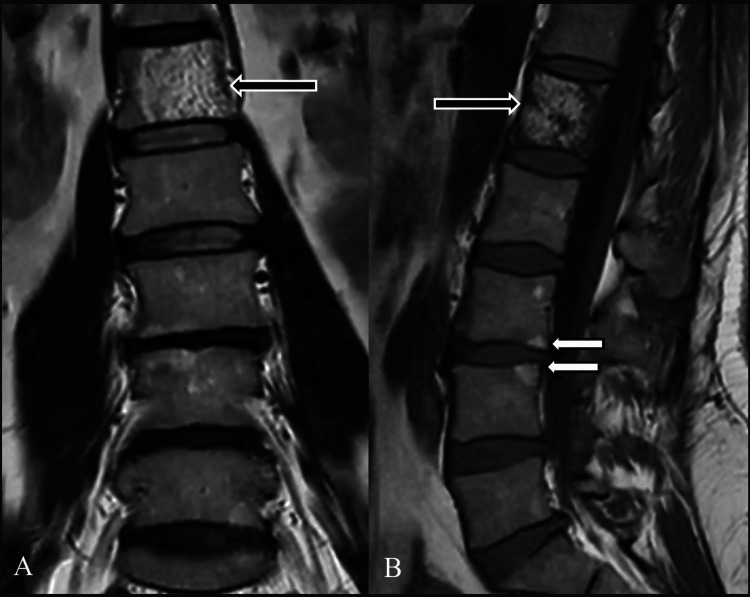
MRI T1 acquisitions in frontal plane (A) and lateral plane (B) of the lumbar spine in a patient diagnosed with SpA. The white arrows point at two vertebral corner fatty infiltration modifications, while the black arrows point at the presence of a large hemangioma occupying approximately 90% of the L1 vertebral body. MRI, magnetic resonance imaging; SpA, spondyloarthritis.
